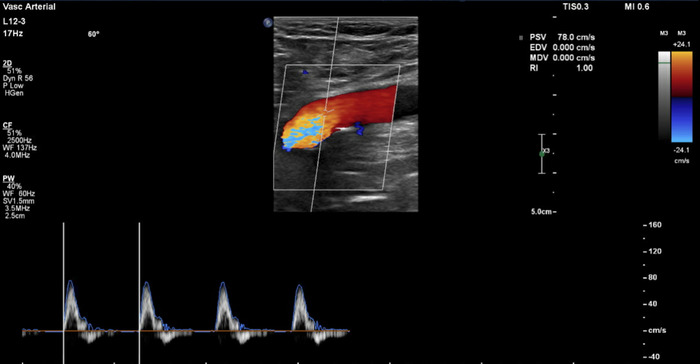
Right lower extremity venous and arterial duplex ultrasound on day 5 of hospitalization depicts near doubling of velocities in the right peroneal artery vs admission, suggesting 50% stenosis. Transition to biphasic waveform suggests mild diffuse atherosclerosis.
